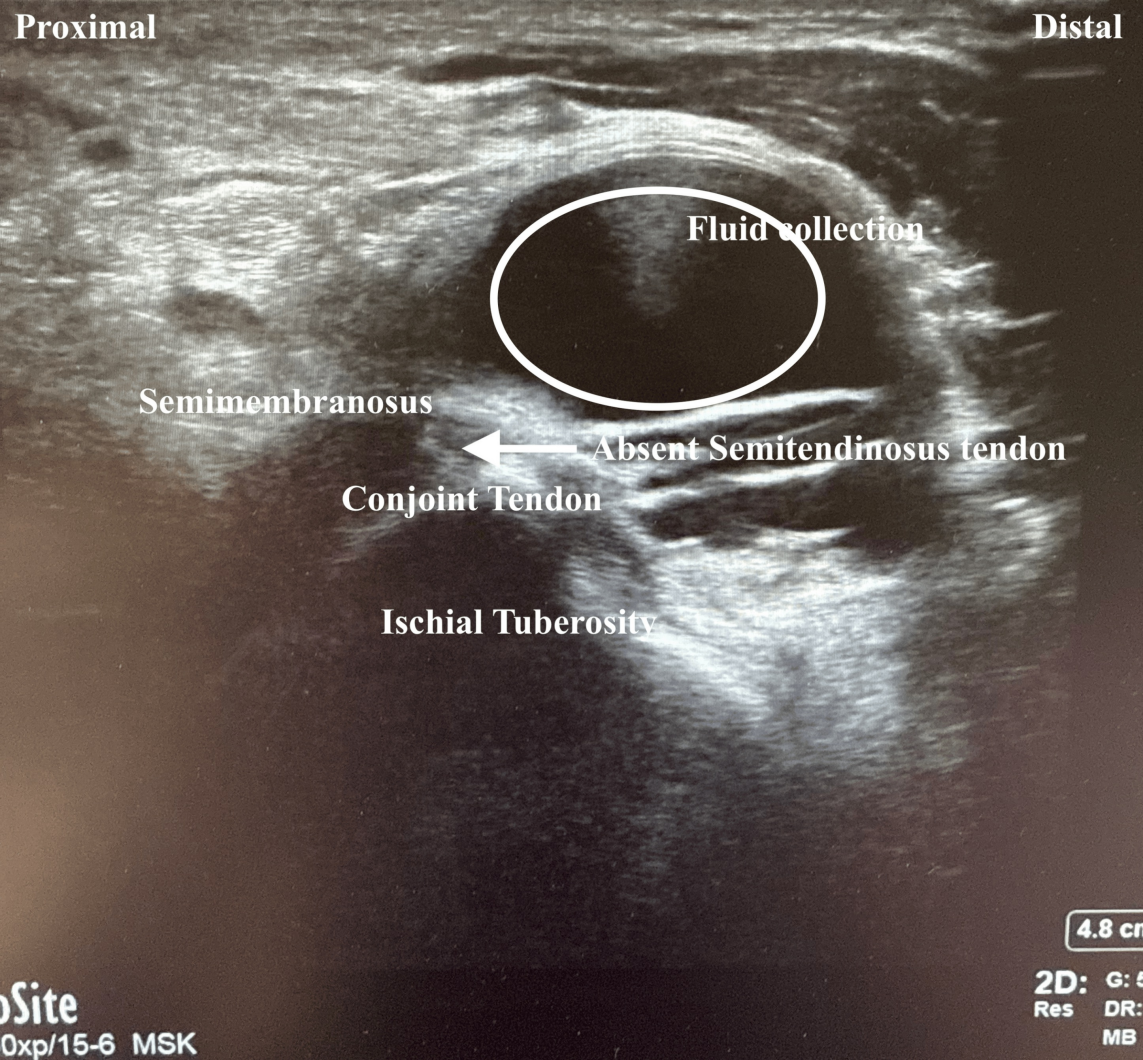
Labeled ultrasound long-axis view of the knee. Note the fluid collection (circle) and absent semitendinosus tendon (arrow).
